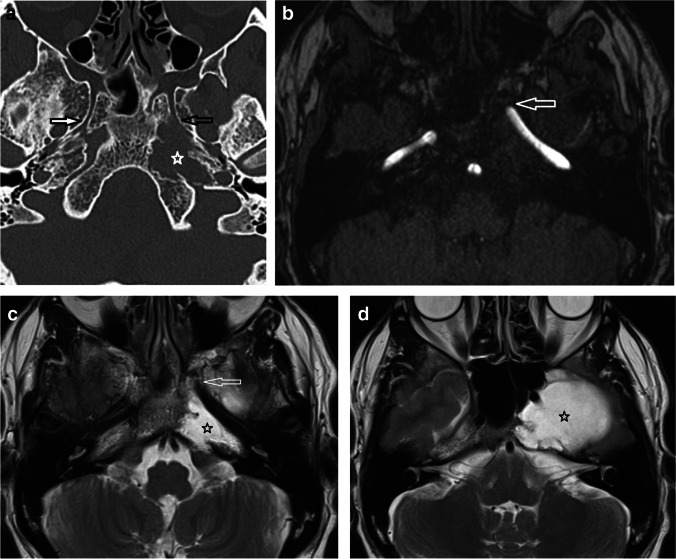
Patient 2. (a) Axial contrast-enhanced CT on bone windows demonstrates the destructive left petroclival chondrosarcoma (white star). The normal right sided VC (filled white arrow) is demonstrated with an intact and normally splayed posterior opening. The left VC has an eroded posterior opening (black open arrow). (b) Axial MRA and (c, d) T2w axial images confirm the location of the pICA (open white arrow) which is displaced in the line of the VC by the more posterior T2w hyperintense chondrosarcoma (black star)
